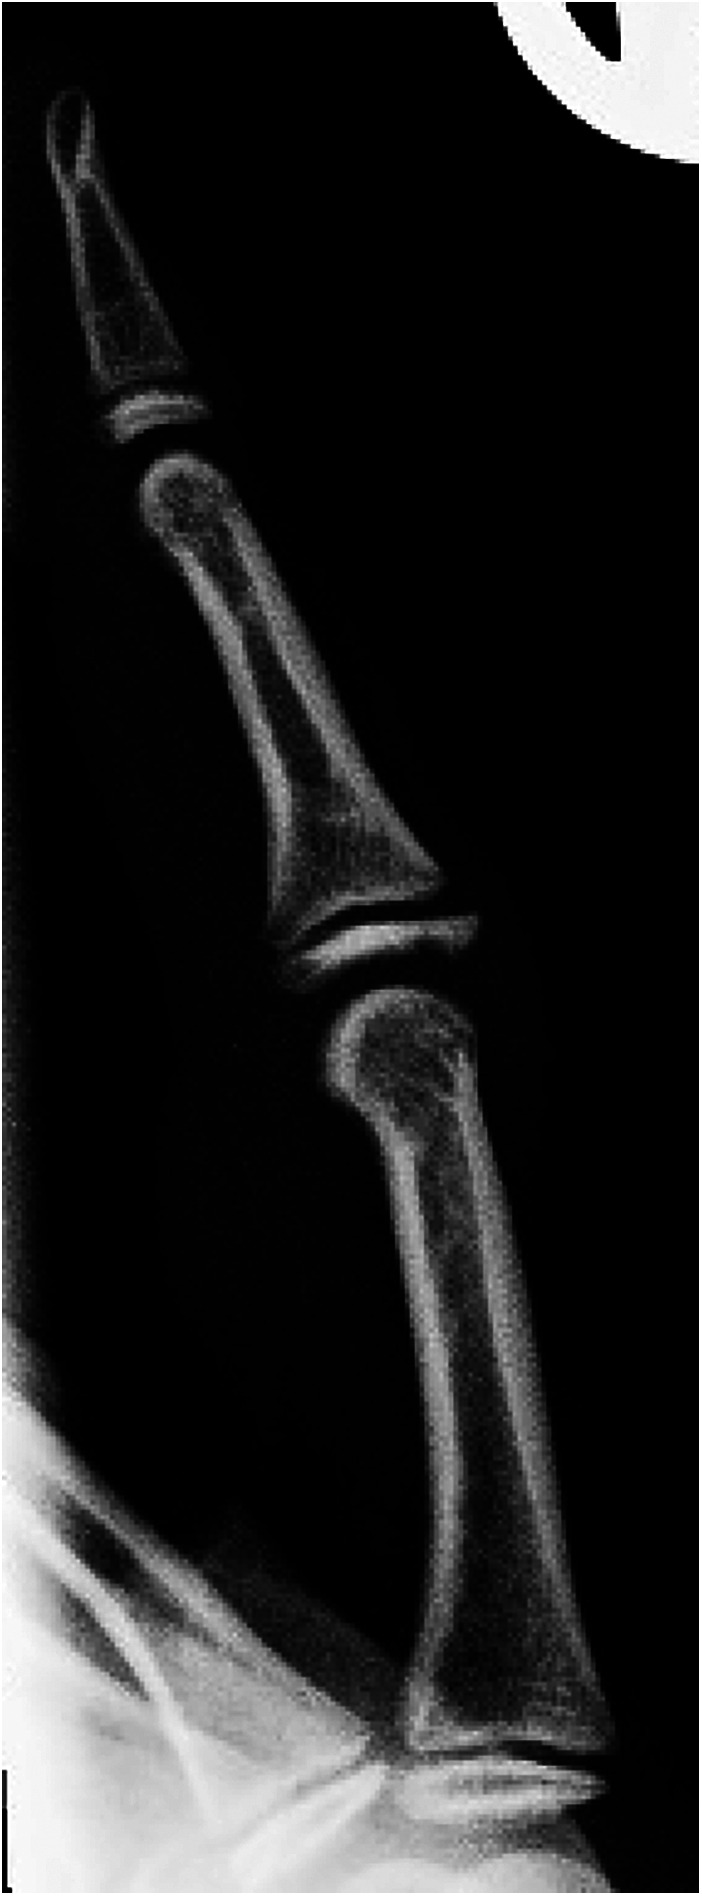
Radiographic sign 1. Dorsal widening of the dorsal middle phalangeal physis with irregularity, fragmentation, and periphyseal osteopenia (4, 8, 9). Physeal widening correlates with hypertrophied chondrocytes extending into the metaphysis, a consequence of disrupted metaphyseal vascular supply (46). Physeal irregularity and fragmentation result, in part, from damage and effacement of the zone of provisional calcification (47). (14 y old girl, right middle finger).
